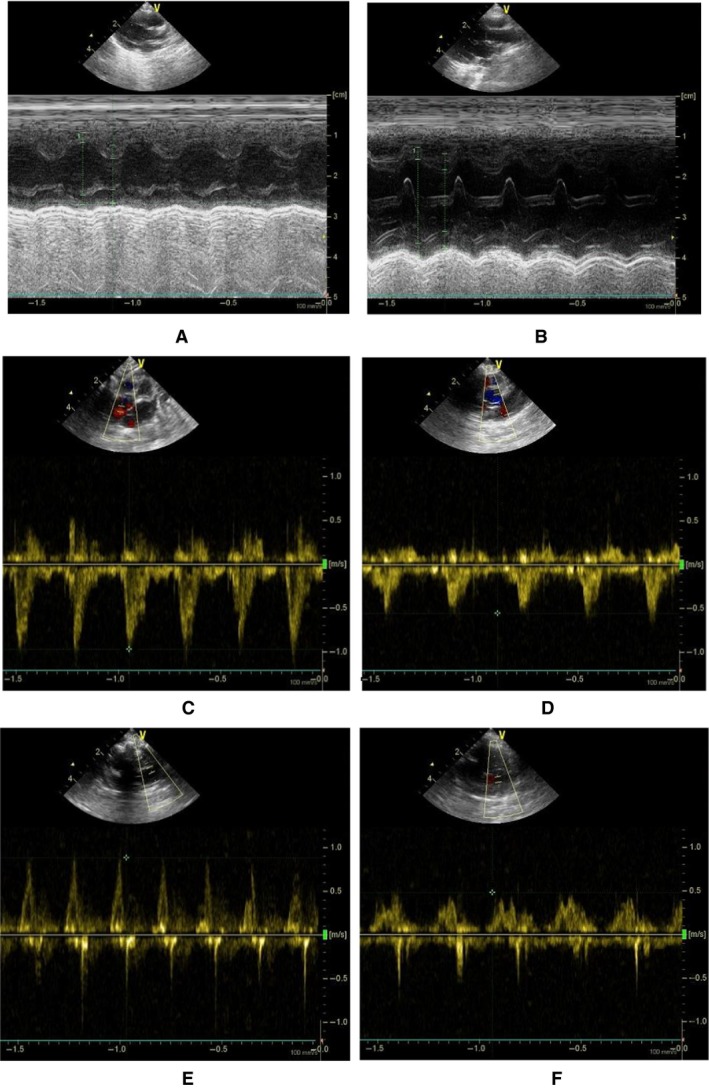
(A–B) Right parasternal short‐axis echocardiographic view with the two‐dimensional guided M‐mode tracing in (A) a sham rabbit and (B) a rabbit of myocardial infarction; (C–D) Subcostal apical five‐chamber echocardiographic view of aortic outflow velocity with the pulsed Doppler tracing in (C) a sham rabbit and (D) a rabbit of myocardial infarction; and (E–F) Subcostal apical four‐chamber echocardiographic view of mitral inflow velocity with the pulsed Doppler tracing in (E) a sham rabbit and (F) a rabbit of myocardial infarction at postoperative 12 weeks.
